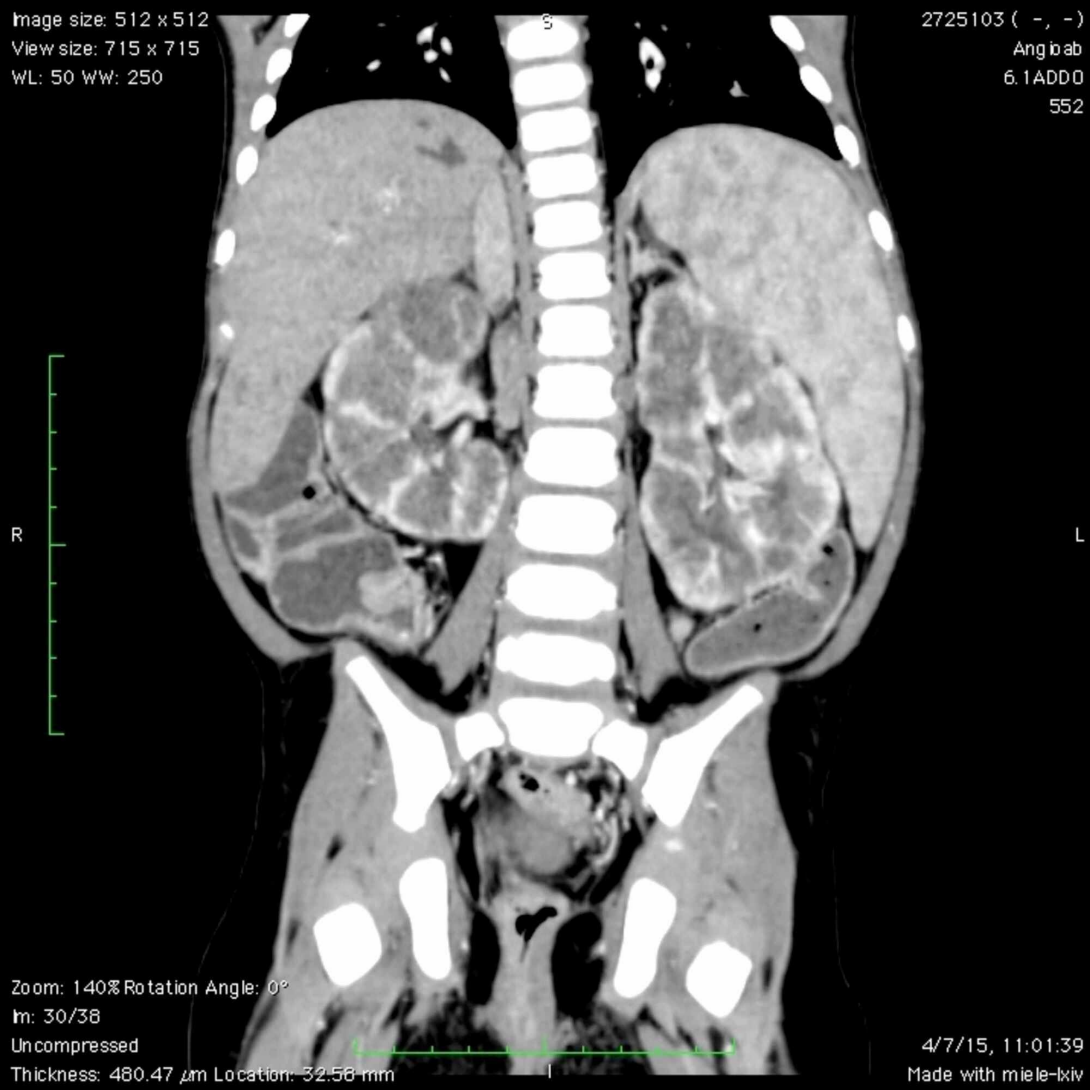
Computed tomography showing polycystic kidneys
